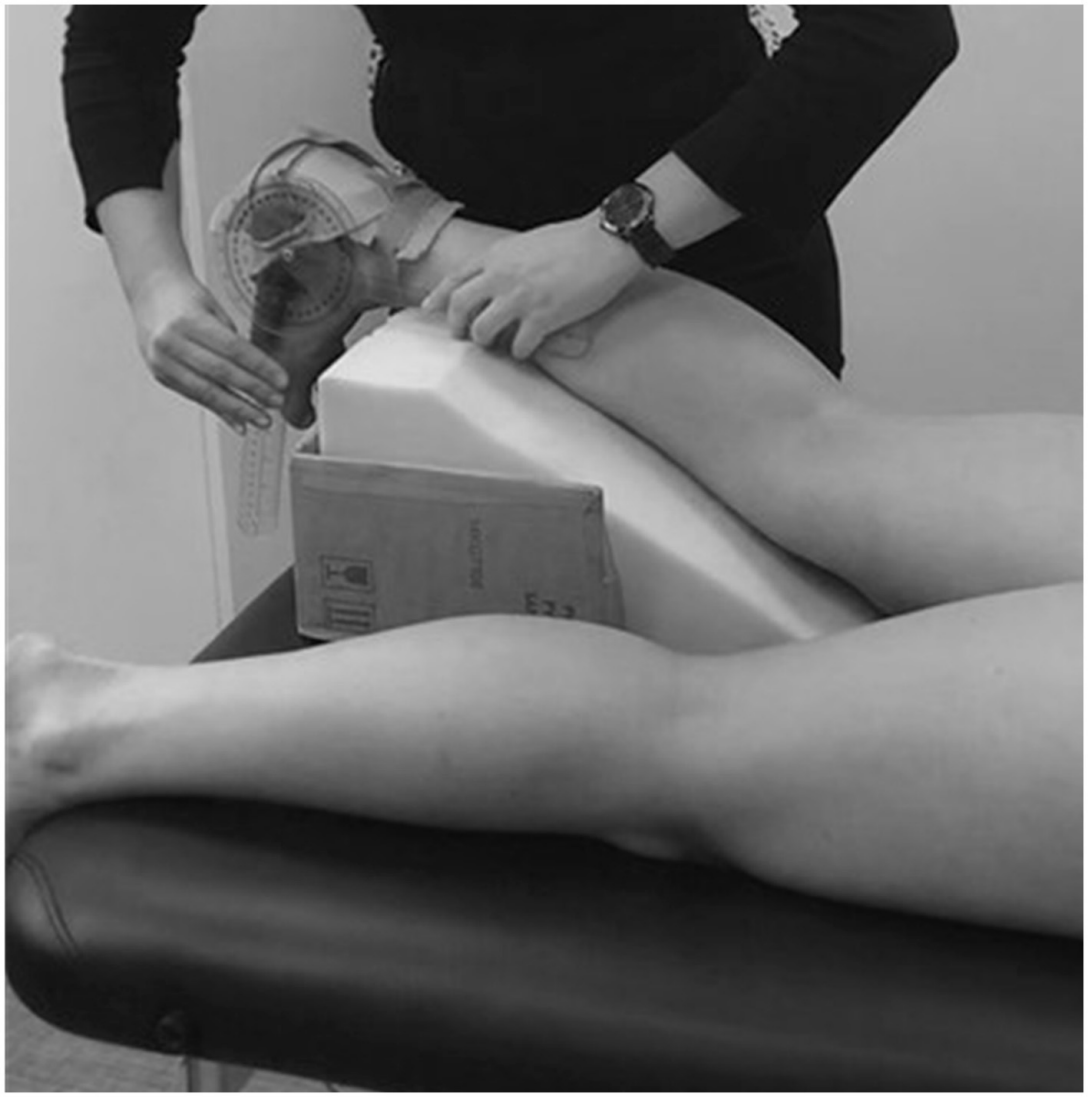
Position of the lower limb during ultrasonography.
